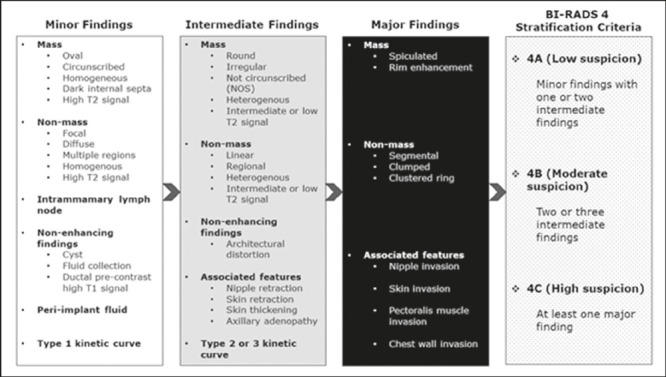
MRI criteria derived from ACR BI-RADS descriptors. In order to be
